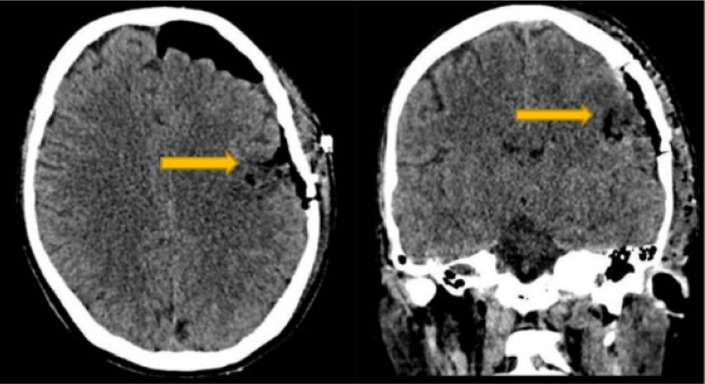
The postoperative brain CT scan ruled out the presence of postsurgical acute complications.
